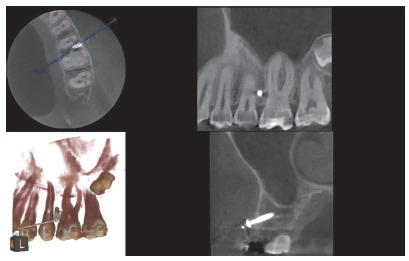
CBCT visualization of three slices and 3D reconstruction.
